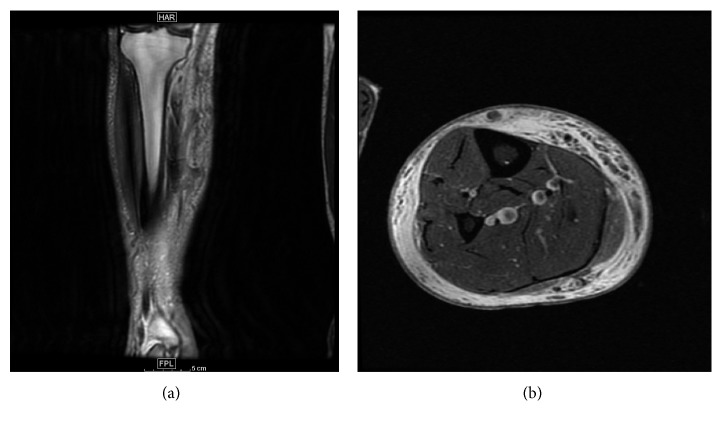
MRI images: (a) coronal T1 MRI Tibia/Fibula; (b) axial PD MRI.
